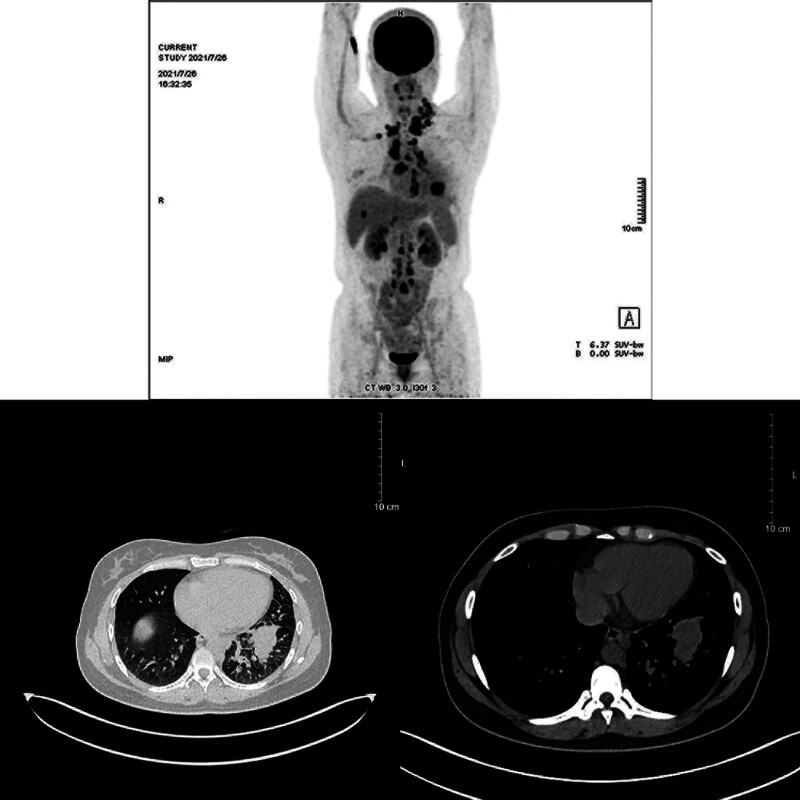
Imaging of PET/CT. Irregular soft tissue mass in the basal segment of the lower lobe of the left lung, considering peripheral carcinoma. Left hilum, mediastinum, left deep cervical space, left posterior cervical triangle, bilateral supraclavicular fossa, bilateral posterior space of the diaphragmatic angle, and retroperitoneum multiple lymph node metastases. Metastatic carcinoma is in the right anterior lobe of the liver. PET/CT = positron emission tomography/computed tomography.
